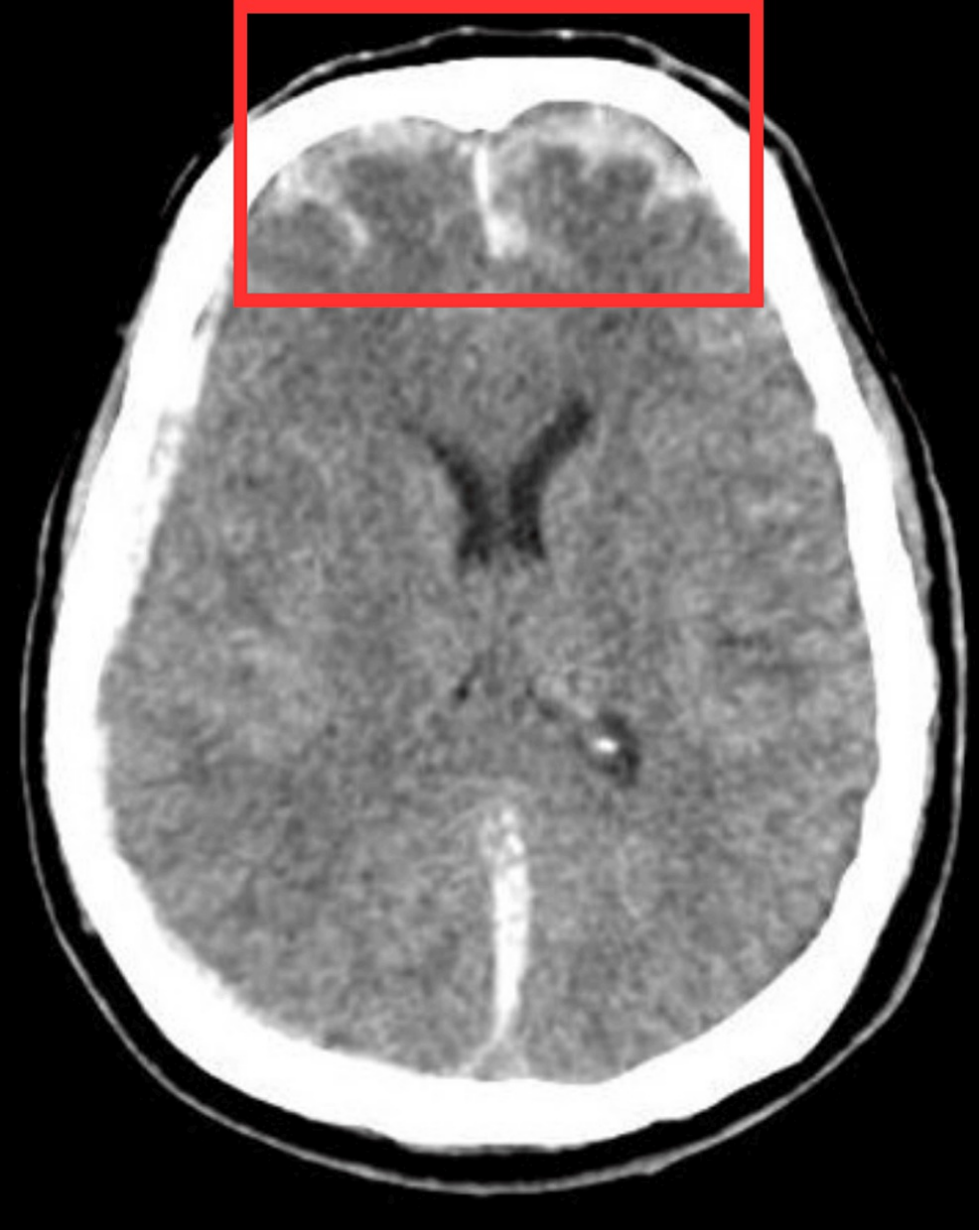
Computed tomography scan of the brain. The red rectangle shows a subdural hematoma on the left side.
